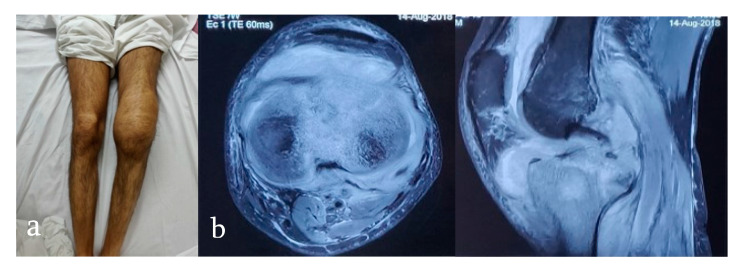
(a) A 28-year-old man presents with pain, gradually progressive swelling in his left knee and restricted left knee movements since the past 3 months. (b) MRI left knee shows synovitis, bone marrow edema with erosions, osteomyelitis with para-articular abscess, and tenosynovitis.
